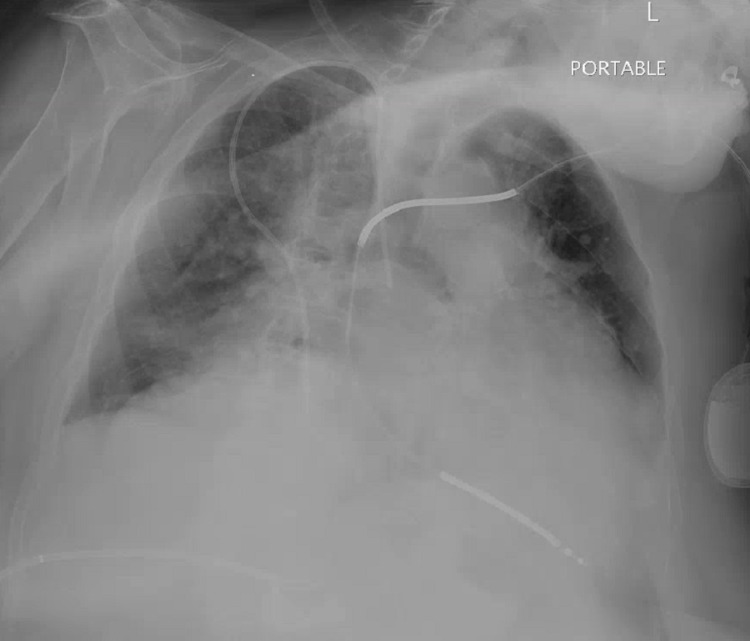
Portable chest X-ray antero-posterior (AP)
